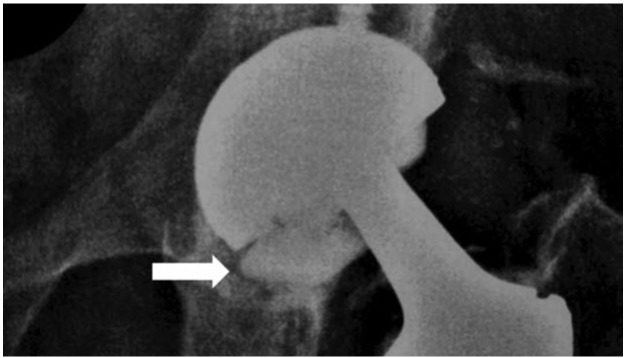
Preoperative radiograph (fractured ceramic liner indicated by arrow).
